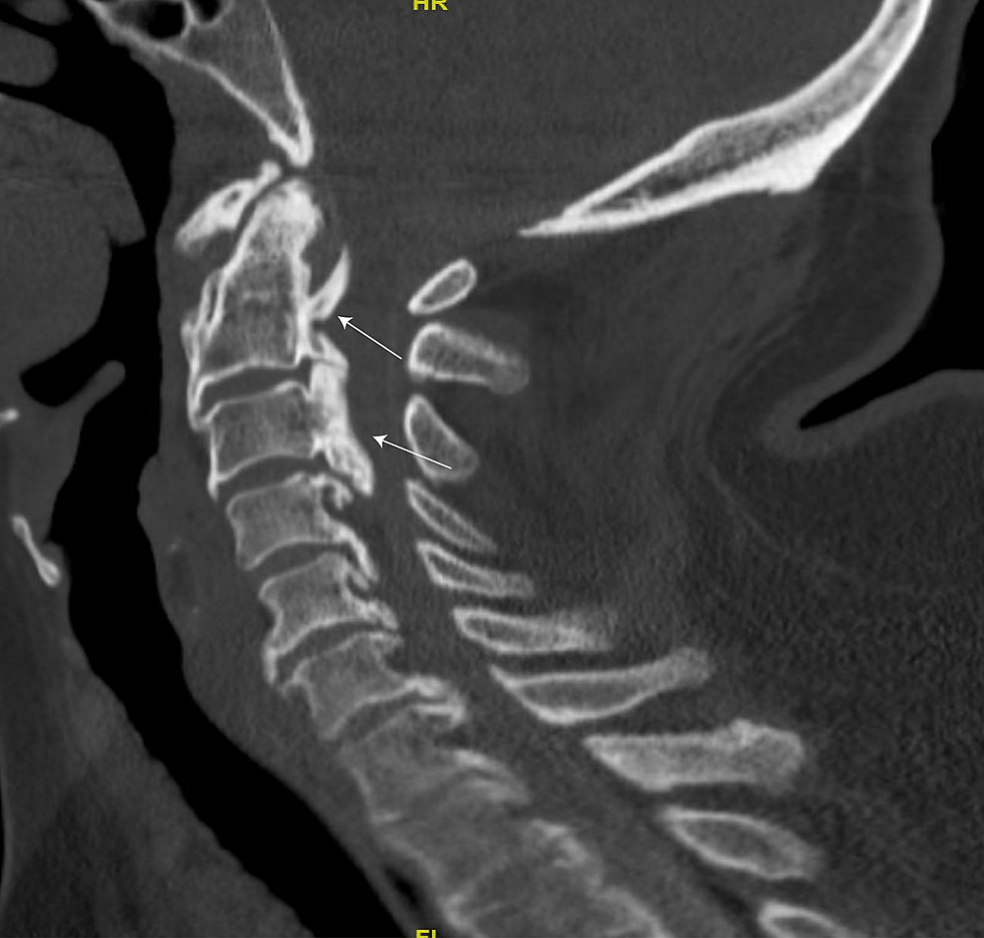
Sagittal CT myelogram demonstrating OPLL from C2-5 in the cervical spine. The arrows indicate few areas of ossification visible on the image slice. CT, computed tomography; OPLL, ossification of the posterior longitudinal ligament
